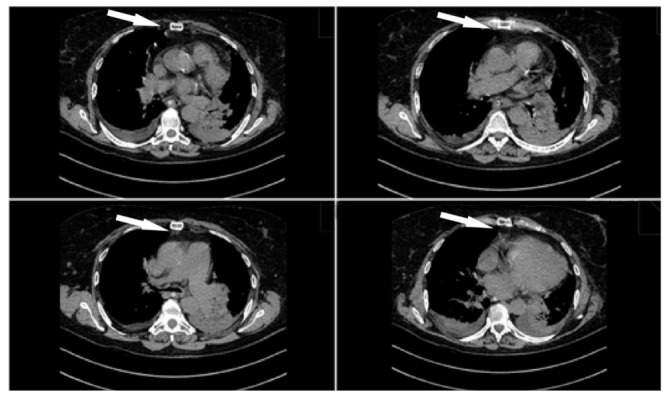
Postoperative chest CT imaging: closed diaphragmatic hernia.
